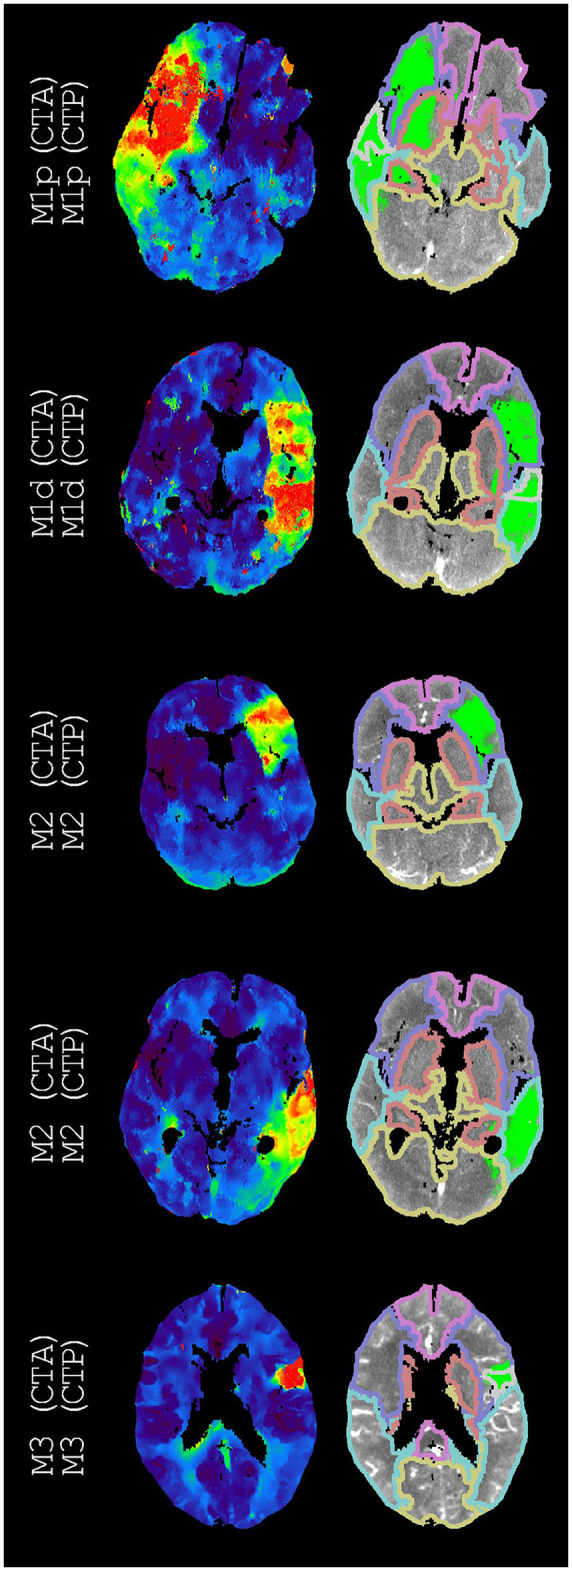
Examples of annotations (CTA) and indications (CTP) that match different segments of the middle cerebral artery. On the left, time-to-peak perfusion maps are shown. On the right, the hypoperfused regions and the downstream regions (according to Figure 1) are shown. For each example, we only regarded the M3 downstream region with the highest intersection-over-union. If displayed, these M3 downstream regions are the superior temporal region, the superior temporal region, and the precentral region (from top to bottom).
